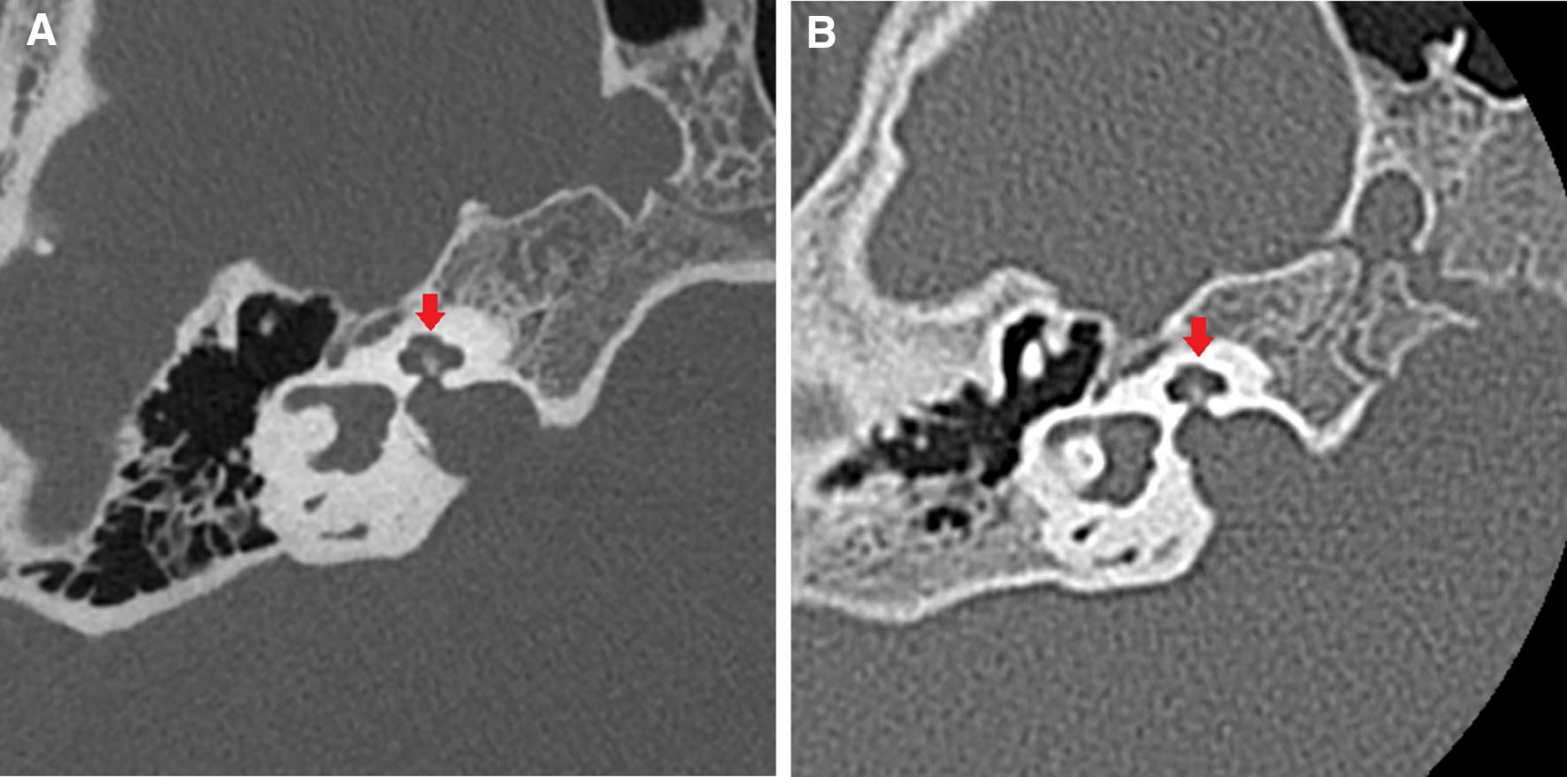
A case of a boy imaged with both EID-CT and PCD-CT for follow-up in his childhood and teenage following hearing loss. A PCD-CT B EID-CT. The internal structures of the cochlea are more clearly delineated by PCD-CT (A, B, arrows)
